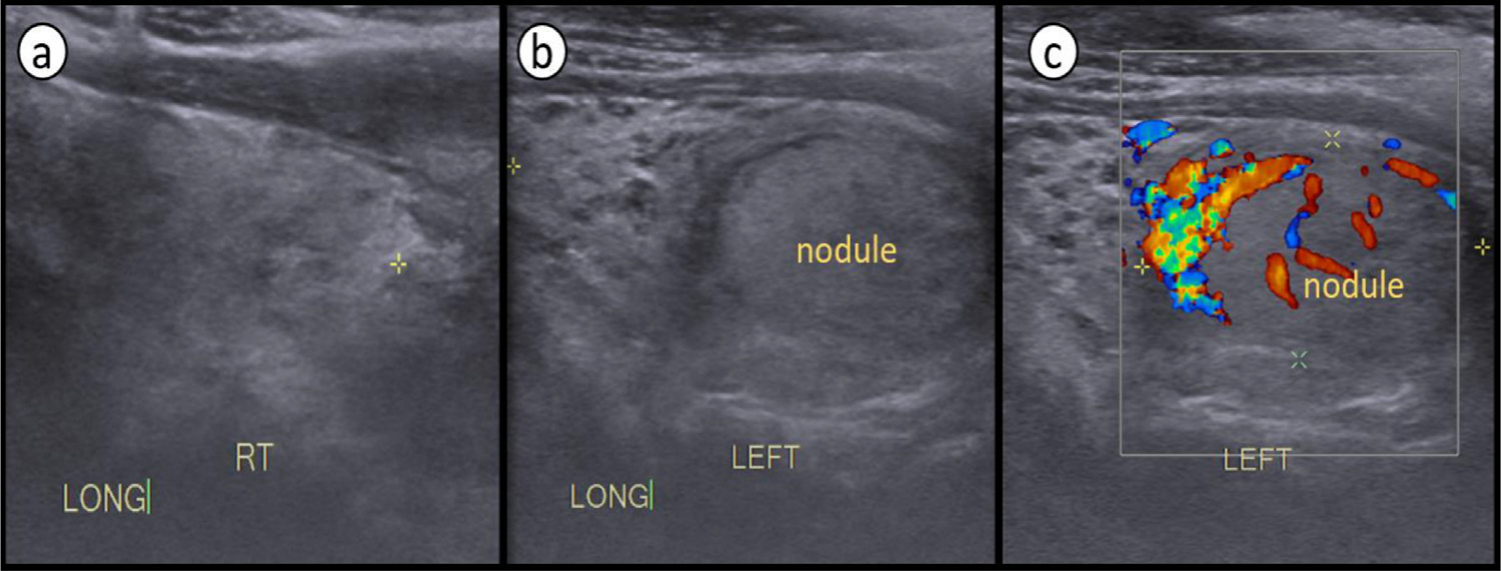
(a-c): Longitudinal ultrasound images of the thyroid lobes. Both lobes showing heterogenous echotexture (a-c). Solitary solid nodule in the left lobe (b,c,C), showing vascularity on doppler ultrasound (c).
